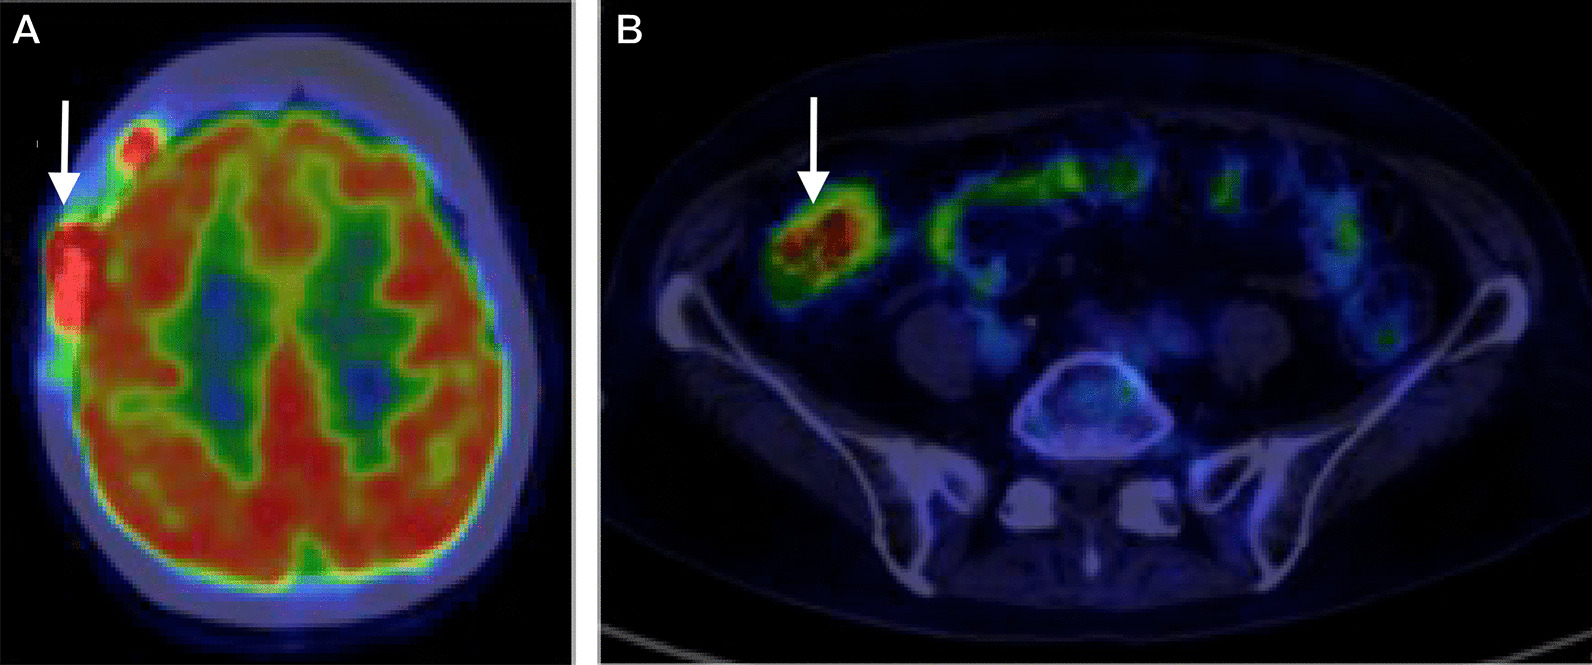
Positron emission tomography–CT images. A Abnormal accumulation consistent with the bone lesion (arrow). B Abnormal accumulation in the large intestine was confirmed (arrow)
